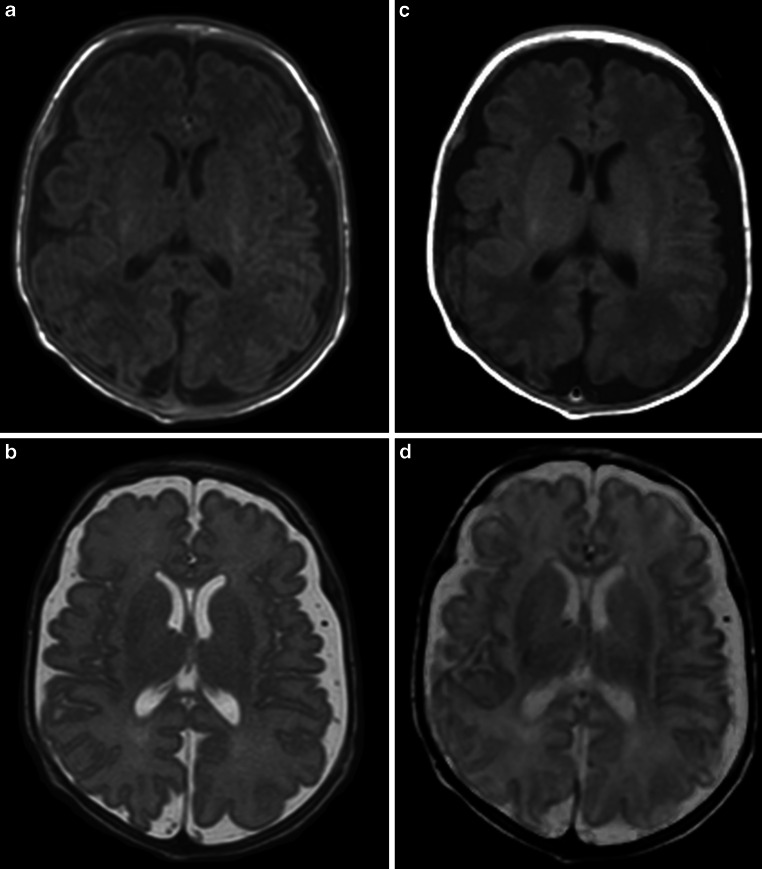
Inconspicuous case: brain of a former preterm infant (24 + 4 weeks). The left column (a, b) shows conventionally acquired T1-weighted (a) and T2-weighted contrasts (b). The right column (c, d) shows T1-weighted (c) and T2-weighted (d) contrasts generated by the MR data post-processing software SyMRI
